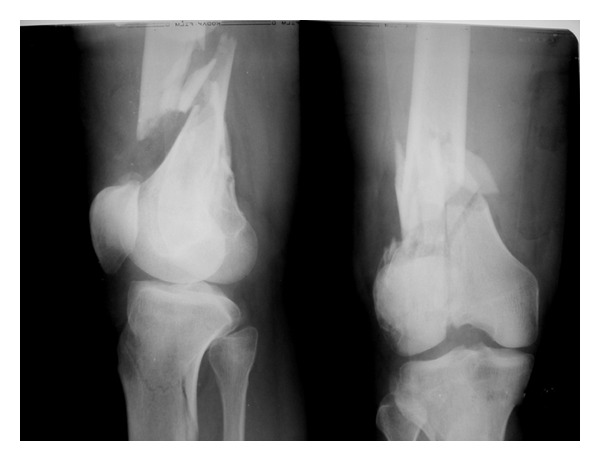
Preoperative X-ray of the right knee AP and lateral views showing fracture distal femur and proximal tibia.
